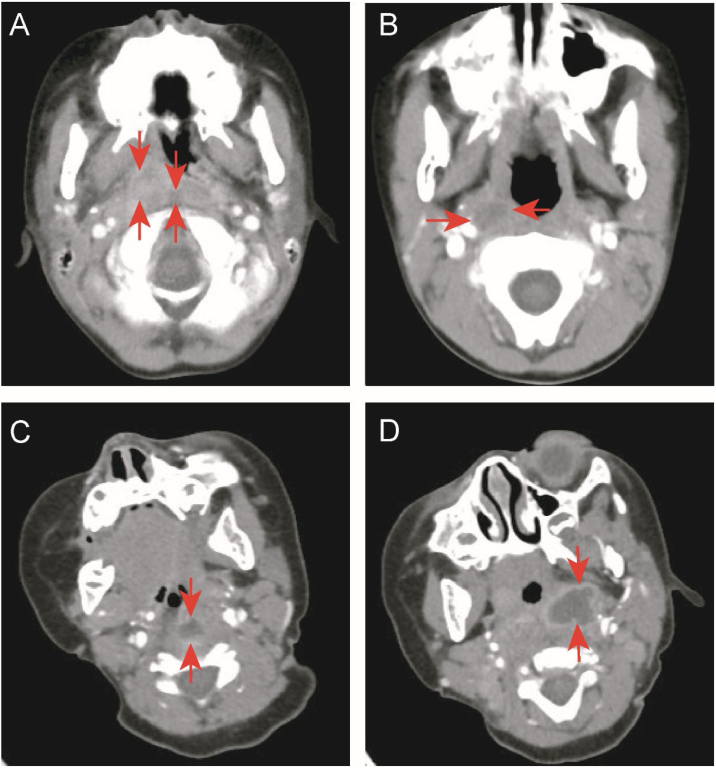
Enhanced neck computed tomography. (A) Retropharyngeal and right parapharyngeal cellulitis (arrowheads). (B) Right parapharyngeal cellulitis (arrowheads). (C) Retropharyngeal abscess with rim enhancement (arrowheads). (D) Left parapharyngeal abscess with rim enhancement (arrowheads).
